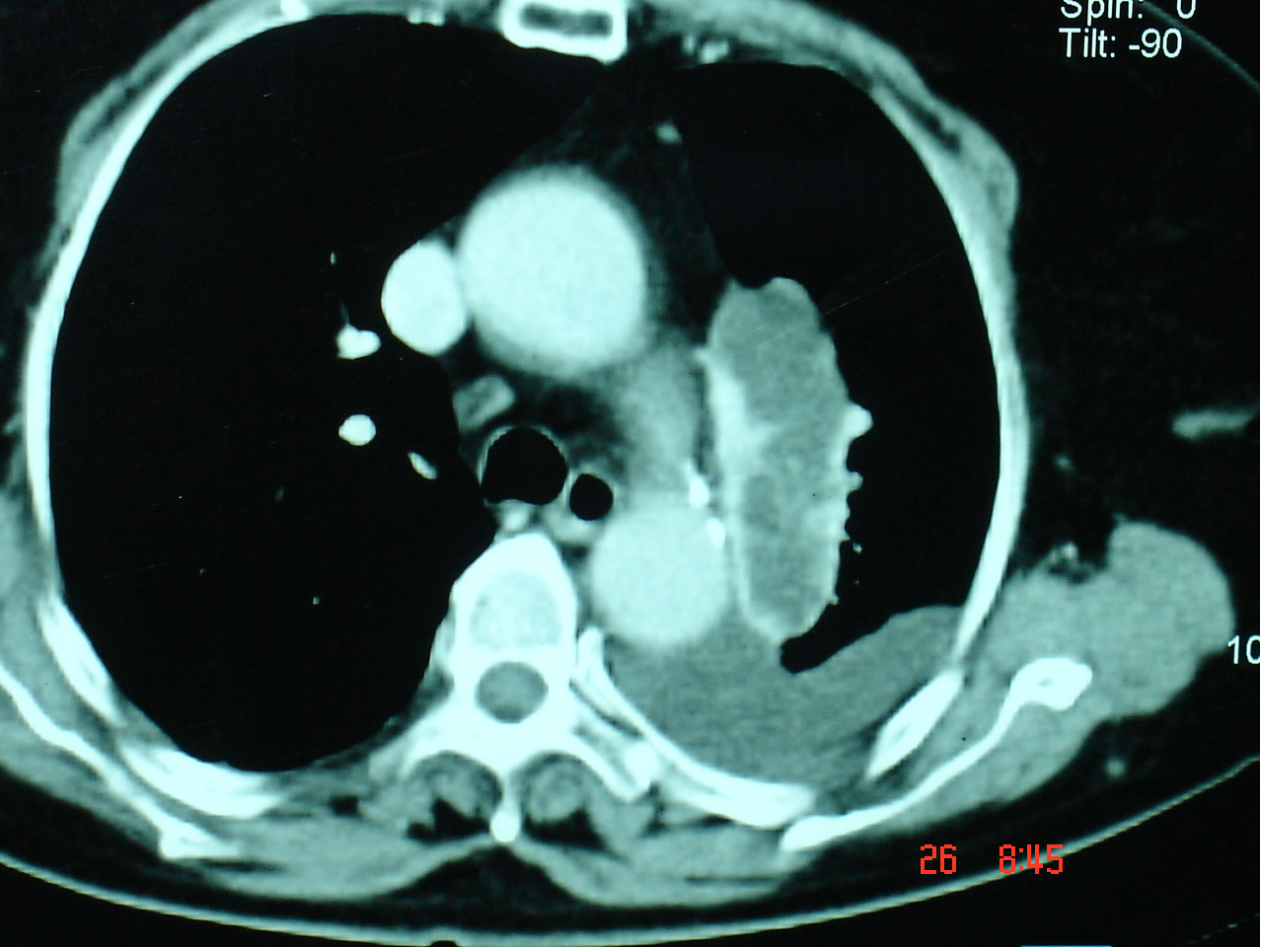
Preoperative computed tomographic scan demonstrated a tumor mass located in the mediastinum next to descending aorta and associated with pleural effusion.
